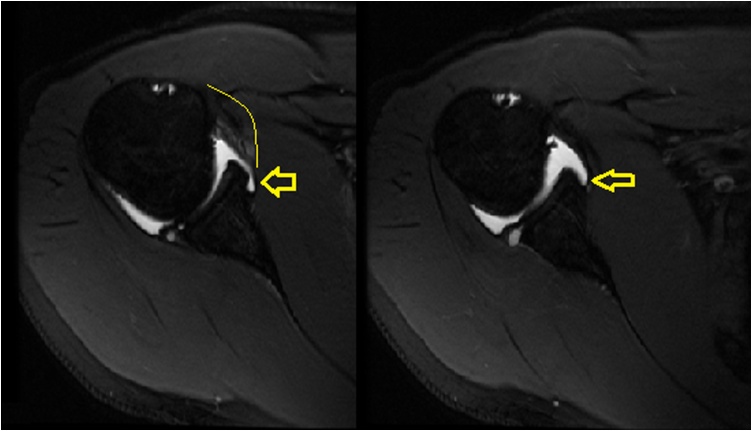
Axial cuts of right shoulder MRI showing posterior labral tear of the glenoid and cyst posteriorly.
